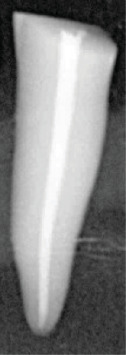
Periapical radiograph demonstrating a root canal system filled with obturation material, indicating adequate canal filling and adaptation.
